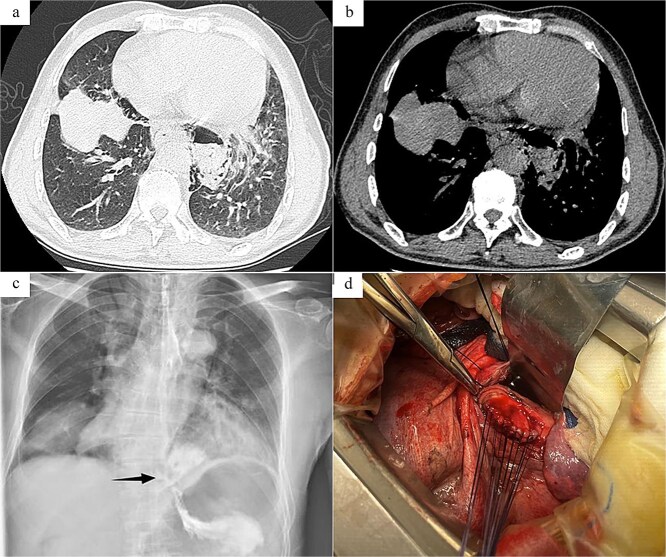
Radiology images and emergency surgery photos of spontaneous esophageal rupture (Case 1). (a and b) Chest and abdomen computed tomography revealed mediastinal emphysema around the lower segment of the esophagus. (c) Esophagus echoendoscopy showed contrast agent leakage into the perihilar region (arrow). (d) Emergency surgery revealed a rupture site located in the lower segment of the esophagus, with a length of about 4.0 cm, and involved interrupted absorbable sutures for full-layer closure of the esophageal perforation.
